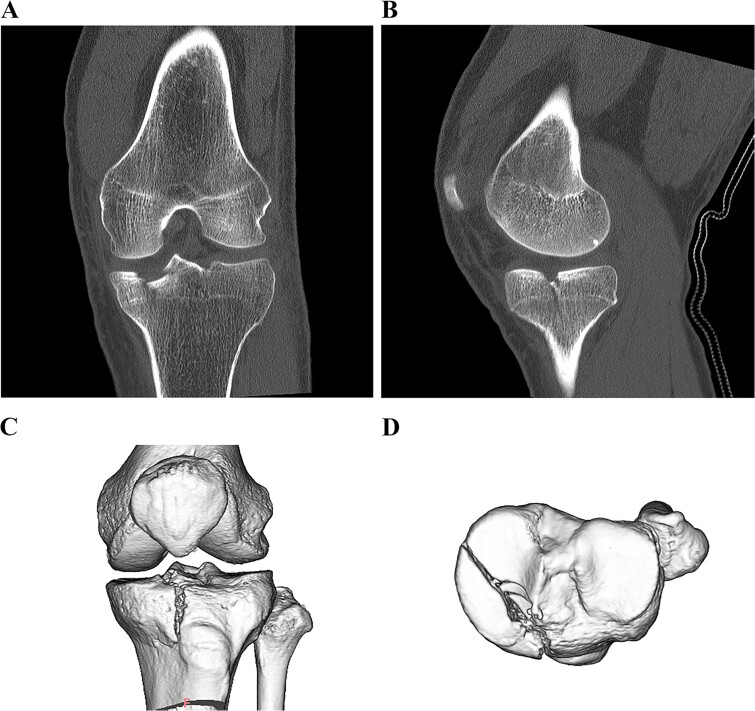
Preoperative CT scan images of the left knee. Coronal and sagittal CT scan images (A, B). 3D CT scan images (C, D). A 5-mm depression in the medial articular surface and column fracture of the tibial plateau are revealed.
